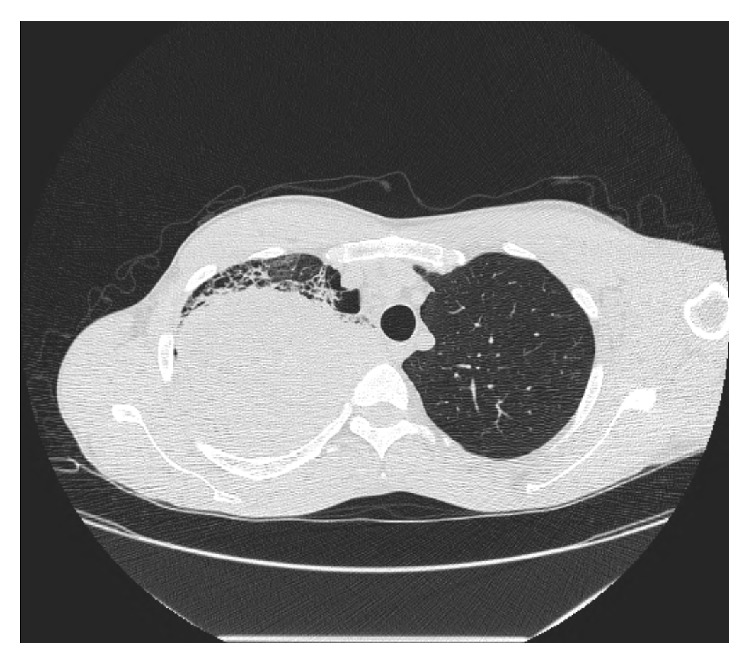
Chest CT scan: Rt upper lobe density.
